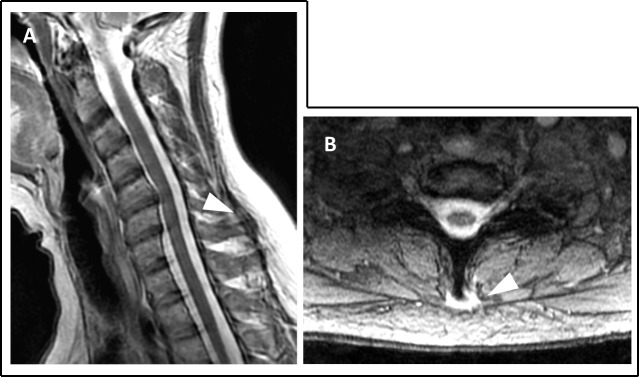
Magnetic resonance imaging (MRI) T2-sequence images of the cervical spine, sagittal, and axial views (A, B) MRI of the cervical spine on both the sagittal (A) and axial (B) views failed to reveal any gross abnormalities involving the soft tissues or neural elements. Retrospectively, no detectable abnormality was evident around the C7 spinous process in the sagittal plane (A; white arrowhead). Although detectable in the axial T2 images, the high signal around the spinous process of C7 (B; white arrowhead) was not striking and was therefore probably overlooked due to being non-specific.
